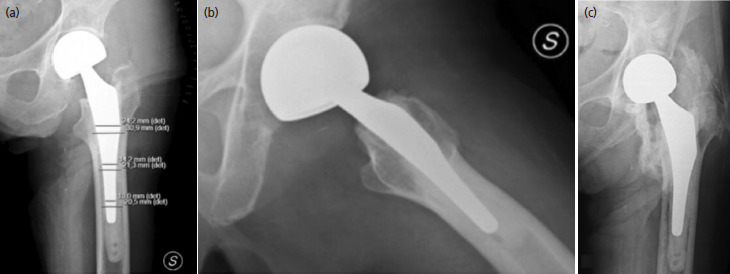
(a) Anteroposterior radiography after hip-hemiarthroplasty through the lateral approach. Example of Canal Fill Index calculation. (b) Post-operative lateral view. (c) Example of heterotopic ossification (Grade 4, second Brooker Classification) 12 months after surgery.
